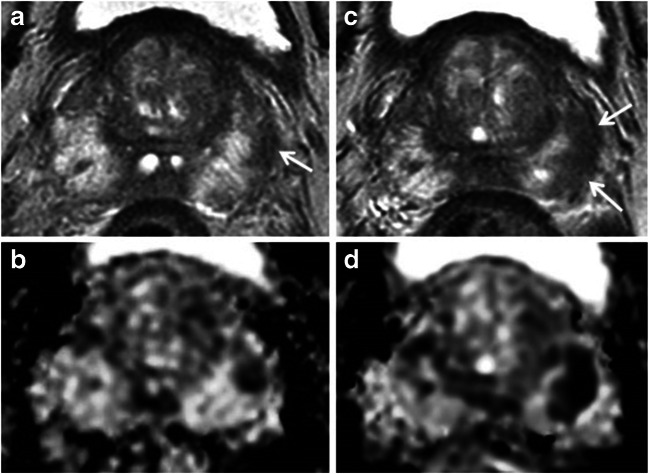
PRECISE score 5. A 68-year-old patient at the time of AS enrolment, with PSA stable at 5.1 ng/ml. Top row: T2 axial; bottom row: ADC maps. a, b Baseline MRI shows a 9 mm × 7 mm lesion at the left base PZ (arrows). Targeted biopsy showed Gleason 3 + 3 = 6, in 2/2 cores, 45%. c, d MRI at 30 months shows the lesion has increased in size to 21 mm × 10 mm, with broad capsular contact and irregularity consistent with ECE. Repeat biopsy showed 3 + 4 disease, 62% of cores. Patient underwent hormone and radiotherapy
